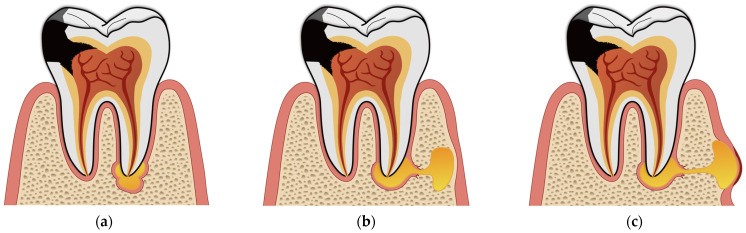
Types of odontogenic abscesses: (a) periapical; (b) subperiosteal; (c) submucosal.
